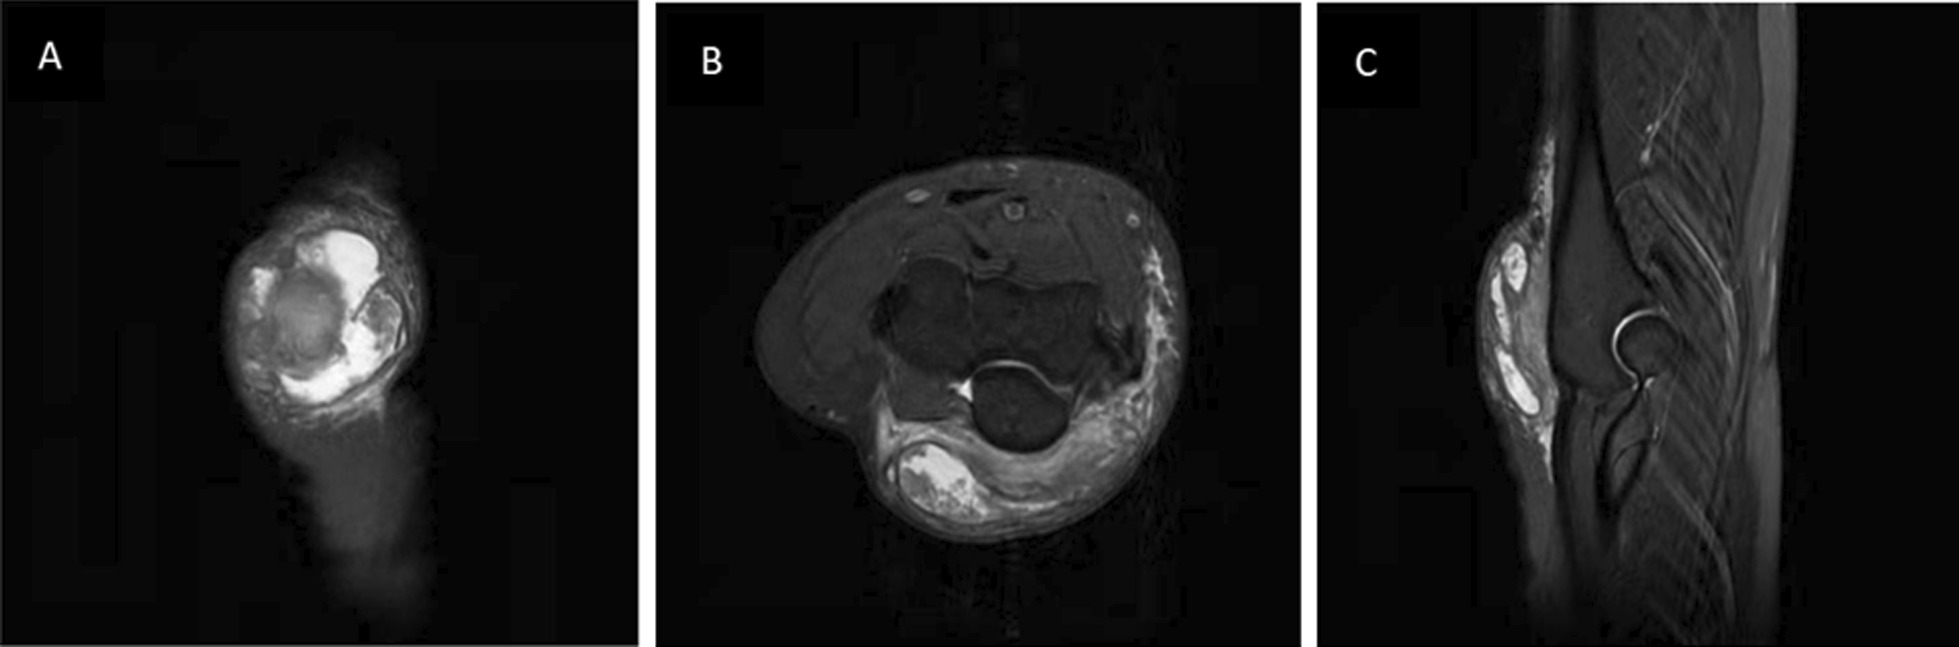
A 28-year-old male patient who underwent an MRI of the elbow joint and showed a huge mass with mixed signals, extensive edema of the surrounding soft tissues, and bony involvement of the adjacent joints. A T2-FS coronal, B T2-FS transverse, C T2-FS sagittal
